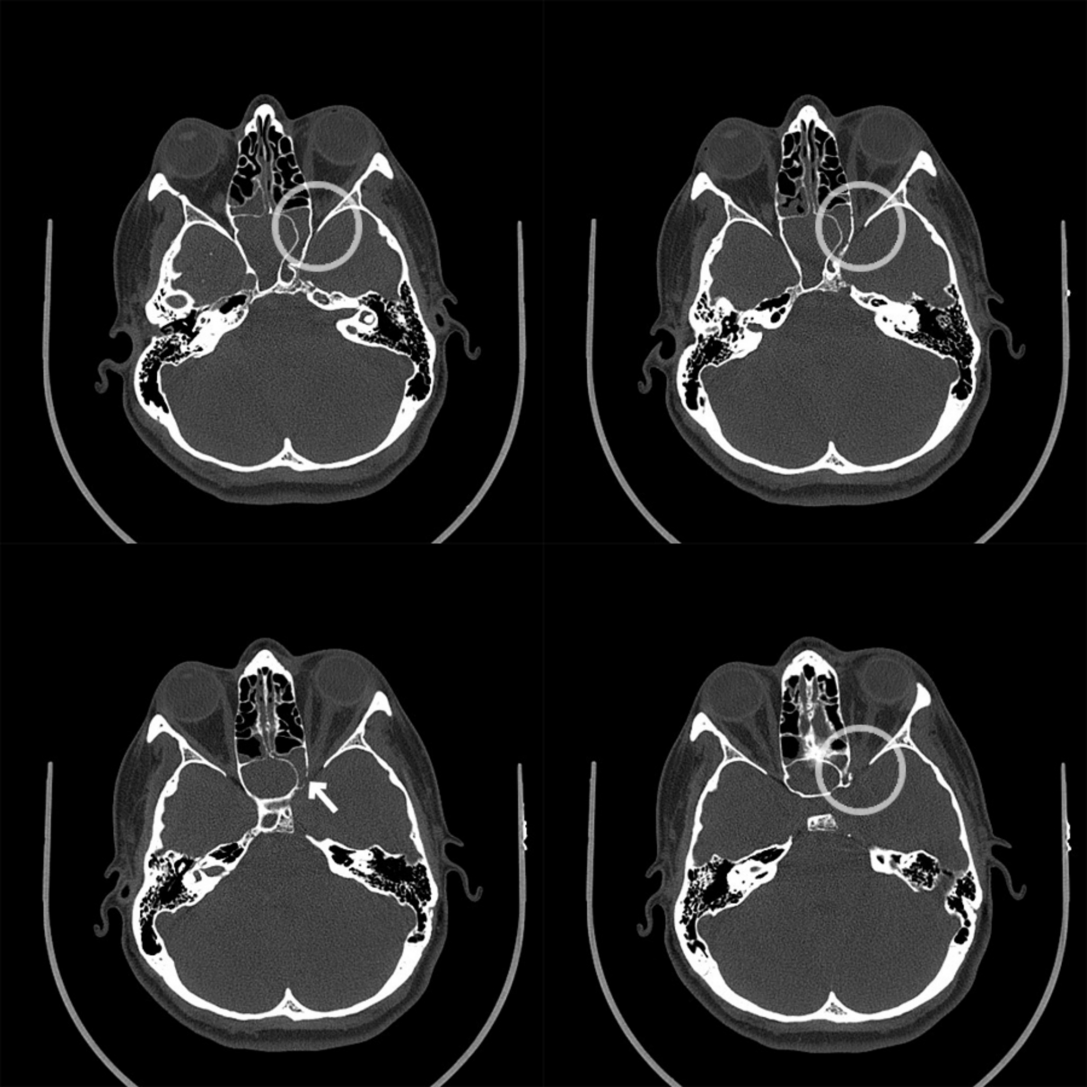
CT Images Progressive invasive sphenoid sinusitis, which invades into the left orbital apex (arrow). Crowding of the left orbital apex demonstrated for which compression of the left optic nerve cannot be excluded. Progressive bone invasion with associated osteomyelitis involving the left anterior clinoid process and the roof of the left orbit with suspicion of adjacent dural thickening effacing the suprasellar cistern, suggesting intracranial extension with associated meningitis. CT: computed tomography
